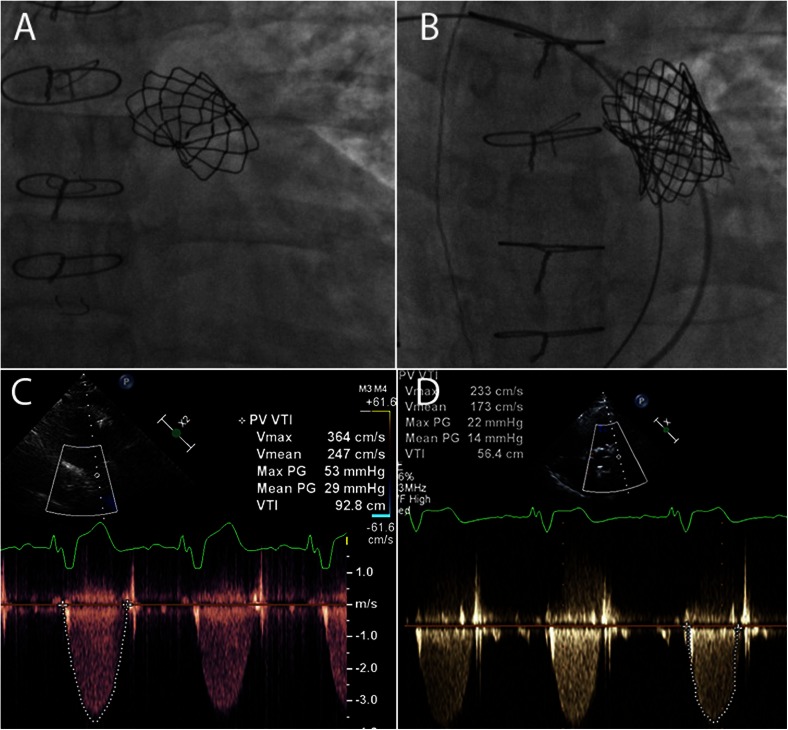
a Antero-posterior (AP) view of a fractured Melody transcatheter pulmonary valve (TPV) within a right ventricular to pulmonary artery homograft. b AP view of a deployed Melody TPV within the previously fractured Melody valve after pre-stenting. c Transthoracic echocardiographic image with continuous wave (CW) Doppler across the fractured Melody TPV demonstrating moderate stenosis (peak velocity 3.6 m/s, peak gradient of 53 mmHg, and mean gradient 29 mmHg). d Transthoracic echocardiographic image with CW Doppler signal across the deployed Melody valve within the previously fractured Melody valve showing significant improvement (reduction in velocity to 2.3 m/s, peak gradient to 22 mmHg and mean gradient to 14 mmHg)
